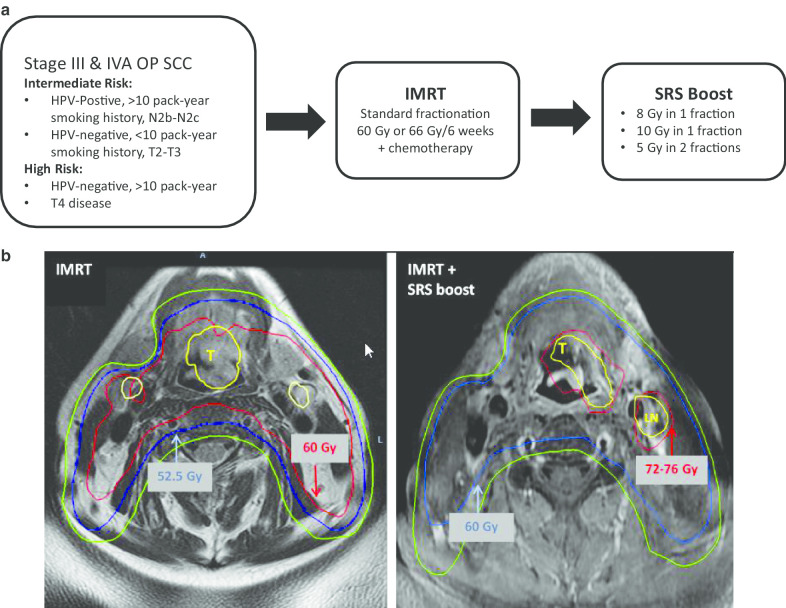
a Patient flow through protocol. b Example IMRT plan prior to any radiotherapy (left) and SRS plan after completion of IMRT (right) overlaid on a T1 post-contrast MRI sequence
